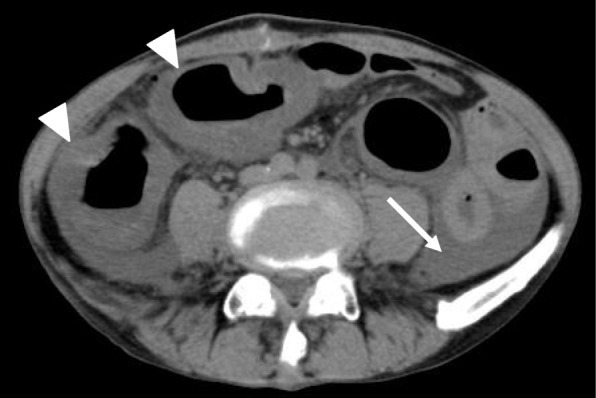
In CT on the 7th postoperative day, ascites (white arrow), colon dilation and colonic wall thickening (white triangle) was evident
